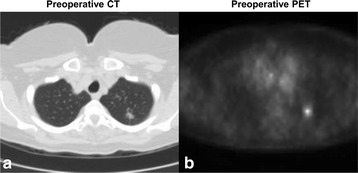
Preoperative CT (a) and PET (b) revealing an FDG-avid nodule in the LUL
